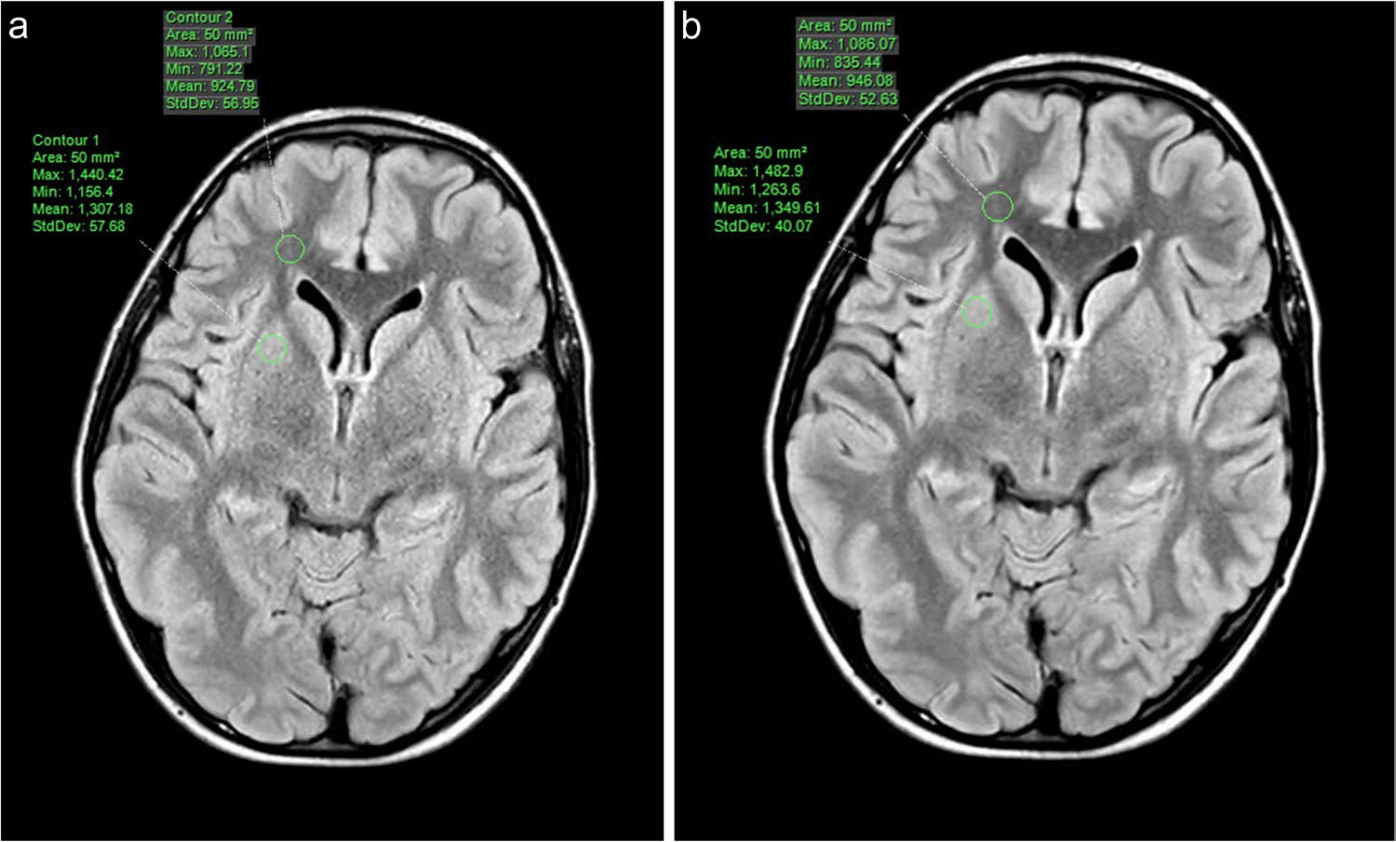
a-b Axial T2W FLAIR brain MR images in an 11-year-old male demonstrating circular ROIs placed in the right frontal white matter and right putamen for signal analysis in the standard reconstruction (a) and AI reconstruction (b) images
